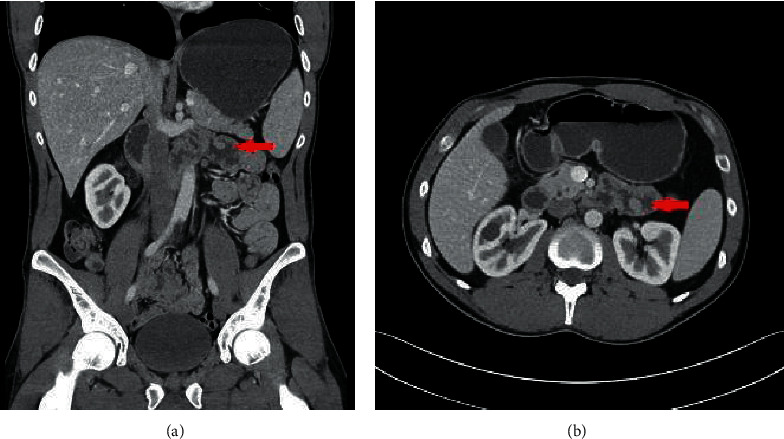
Coronal (a) and axial (b) computed tomography enterography of the abdomen and pelvis with contrast demonstrating a 0.8 cm jejunal polyp in the proximal jejunum just past the ligament of Treitz.
